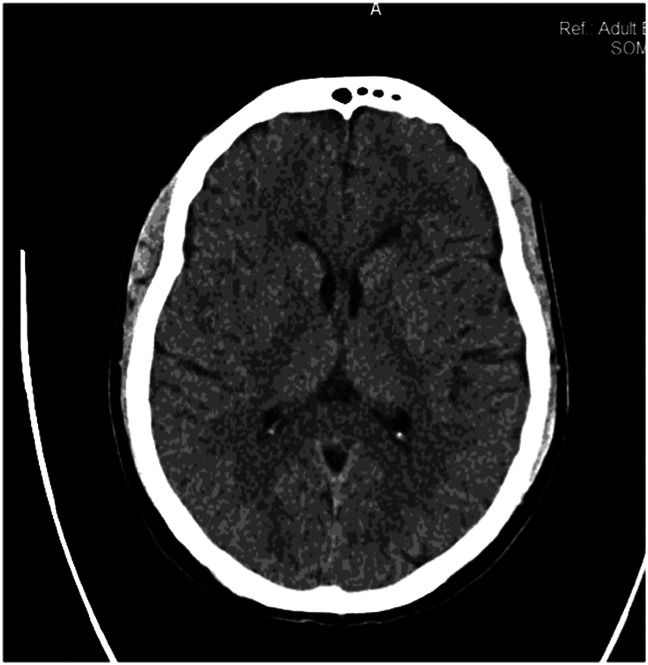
Axial noncontrast CT of the brain showing no abnormalities. CT = computed tomography
